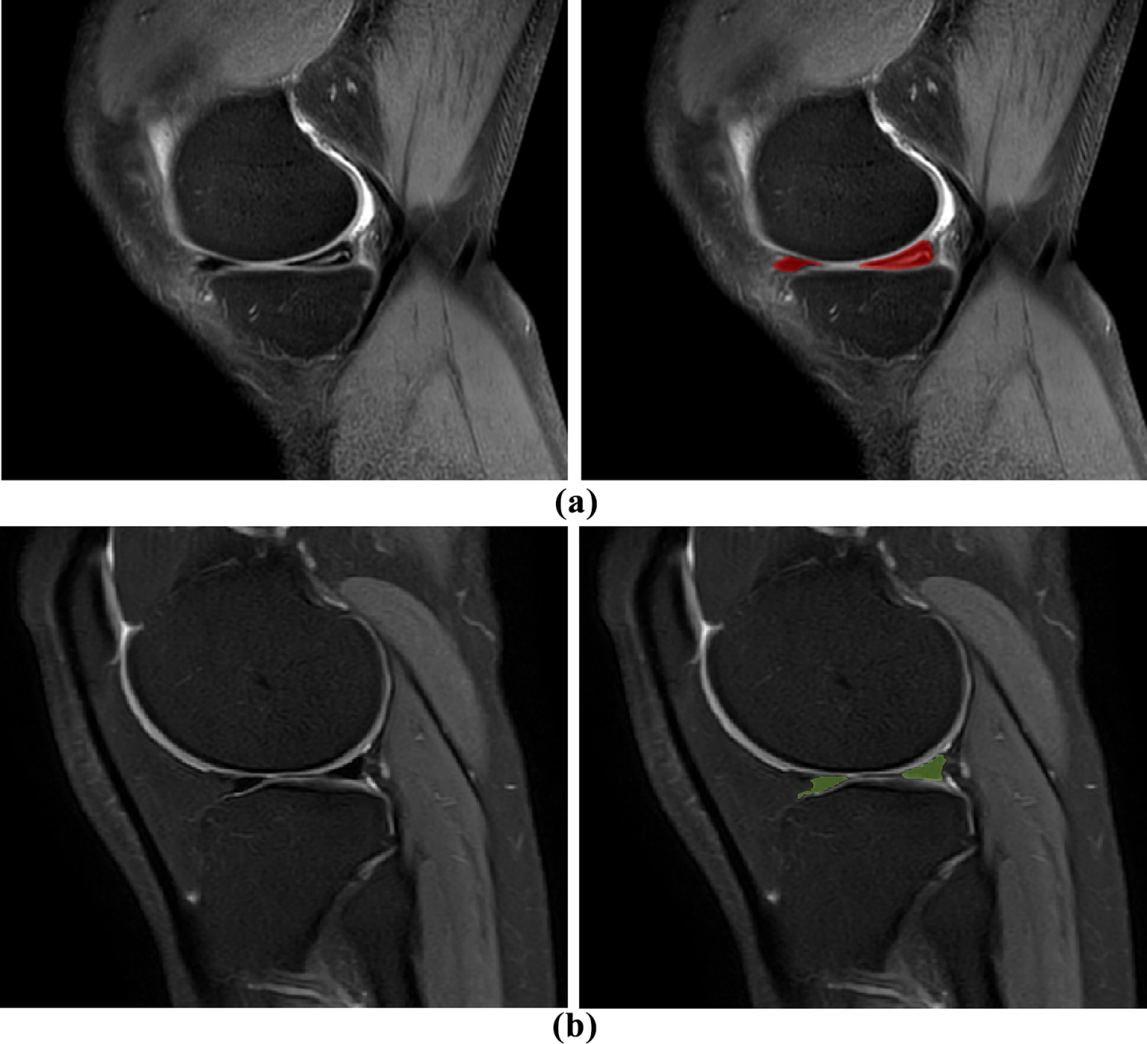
MRI PDW images of the right knee joint. a Tear of the posterior horn of the medial meniscus of the right knee. b None-tear meniscus of the right knee joint; the red and green areas are manually segmented meniscus images
